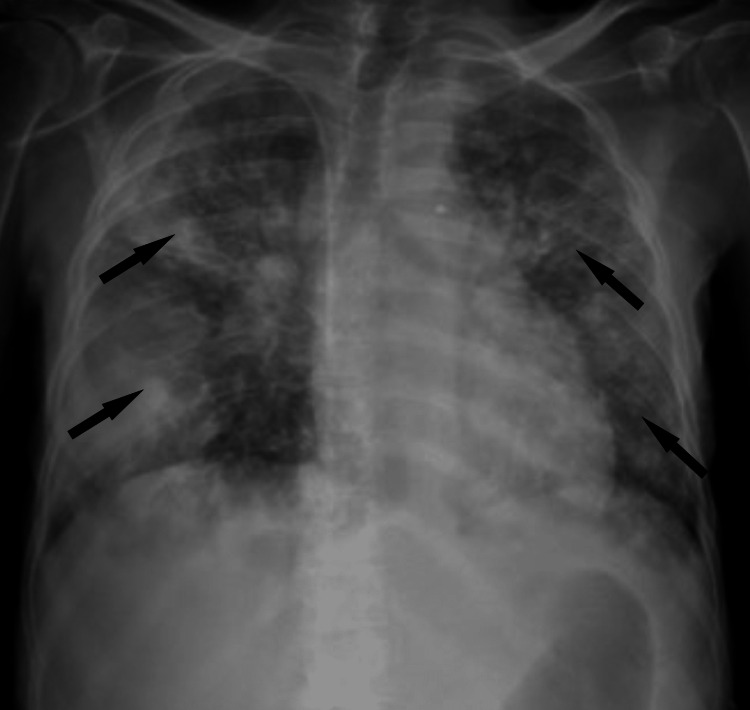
Chest X-ray. Apical to caudal interstitial infiltrates bilaterally (arrows).
